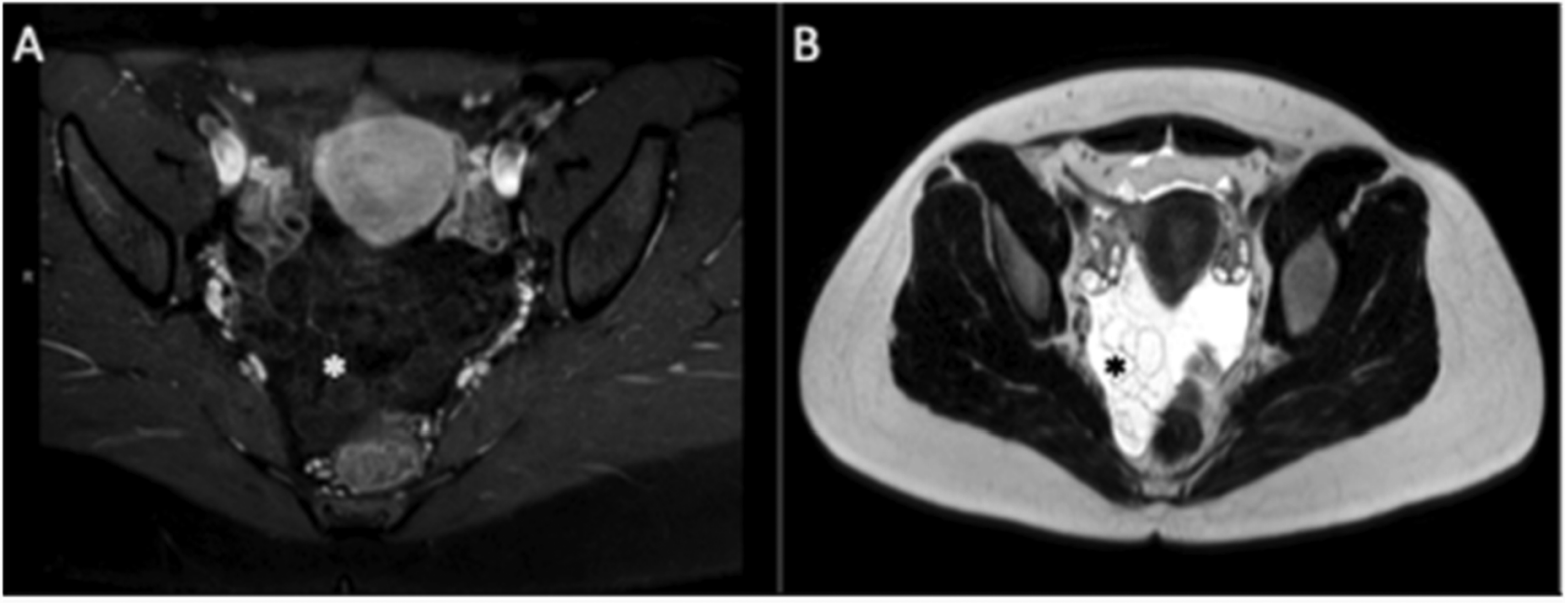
Multicystic mass (star) located in the pelvis with a decreased signal on T1-weighted image (A) but an increased signal intensity on T2-weighted image (B).
